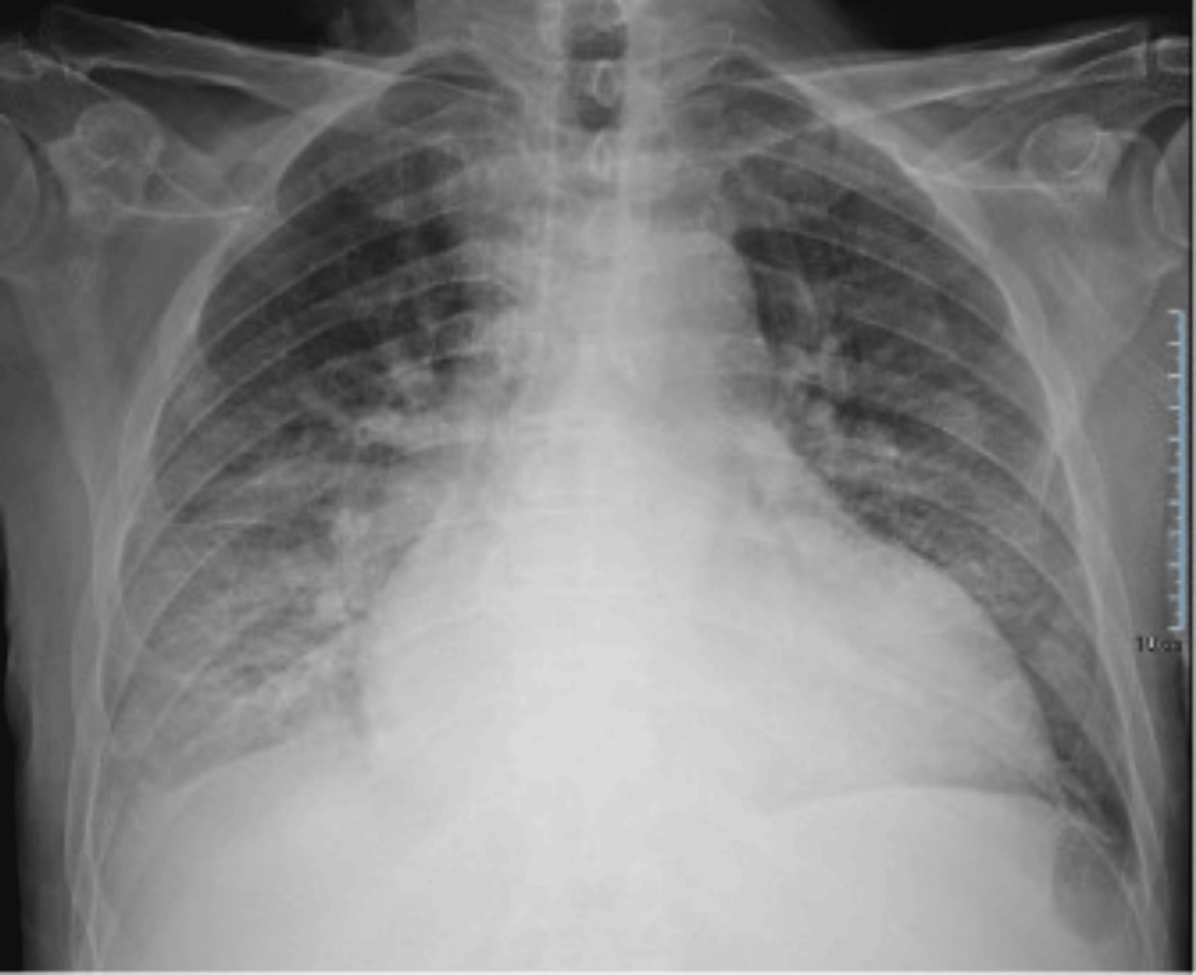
Chest X-ray at admission showing cardiomegaly with signs of pulmonary venous congestion.
